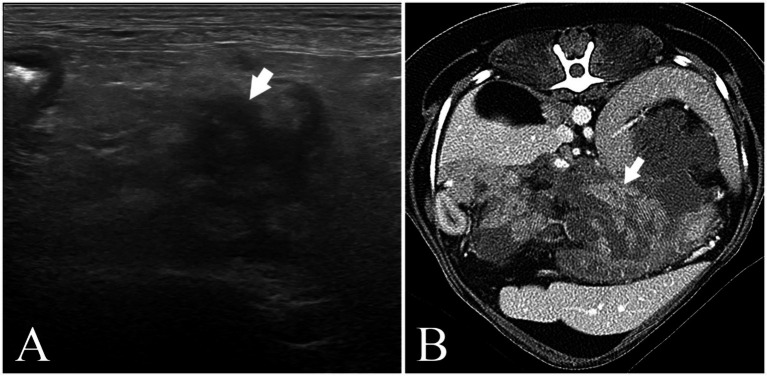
Abdominal ultrasound image (Philips Affiniti 50G) and abdominal CT scan image (Canon Aquilion Lightning) (A) (Pomeranian, 9y, CM) abdominal ultrasound image. The pancreas (arrow) is swollen, irregularly marginated, hypoechoic, and the surrounding fat tissue is hyperechoic. His cPL (1,252➔176ng/mL) and CRP (9.2➔1.6 mg/L) decreased after MF-STEM cell treatment. His pancreatitis recurred on the follow-up. (B) Abdominal CT scan image. (Bichon Frise, 6y, SF) The pancreas (arrow) is swollen, hypoechoic, and has an irregular margin. His cPL (324➔85ng/mL) and CRP (2.6➔0.1 mg/L) decreased after MF-STEM cell therapy. Furthermore, his bilirubin (1.8➔0.13 mg/L) decreased.
